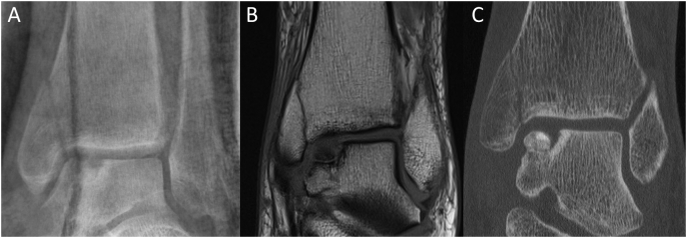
A: Anteroposterior (AP) ankle radiograph after trauma: medial malleolar fracture without displacement; bone defect at the joint line of the medial talus shoulder; B: MRI of the ankle; recent fracture of the medial malleolus and a 4th grade OD at the medial shoulder of the talus with a defect size of 9.4 × 5.7 mm; C: associated CT scan.
